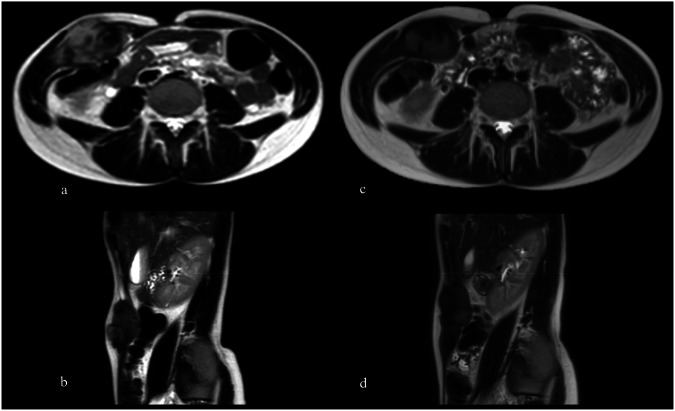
Response assessment with M-RECIST in patients with non-contrast MRI (progressive disease). For patients without adequate contrast-enhanced acquisitions at the latest available follow-up, tissue responses were assigned after a combined evaluation of T1, T2 and DWI changes after treatment, together with a retrospective evaluation of contrast-enhanced acquisitions at all available priors. T2-weighted images before (a, b) and after PC (c, d). Due to the persistence of pathological tissue, the case was classified as progressive disease (PD) with both RECIST 1.1 and M-RECIST
